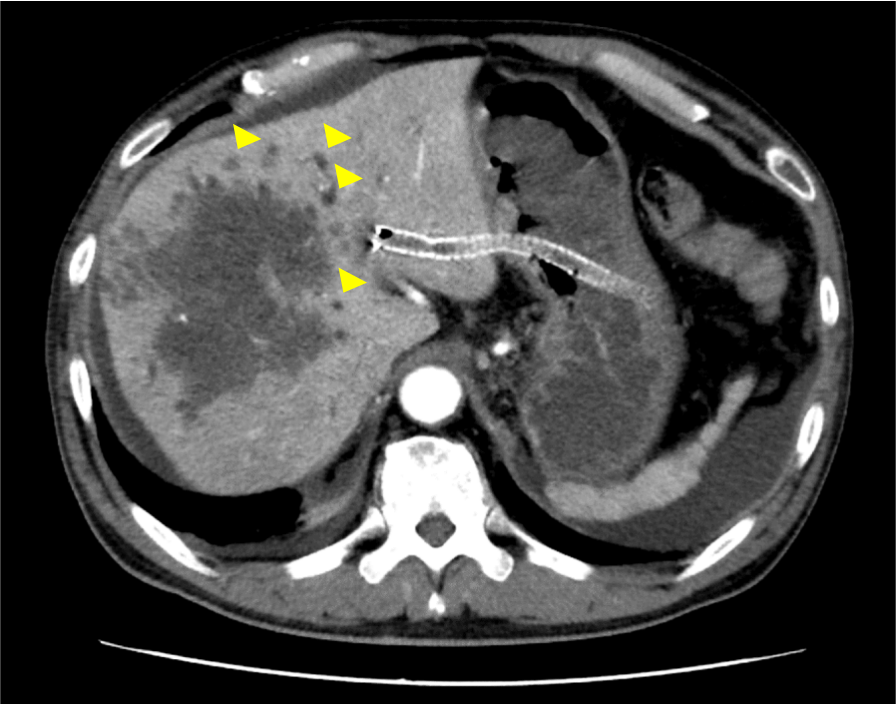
Computed tomography showing hepatic metastases and dilated peripheral bile ducts (yellow triangle) and hepaticogastrostomy stenting
